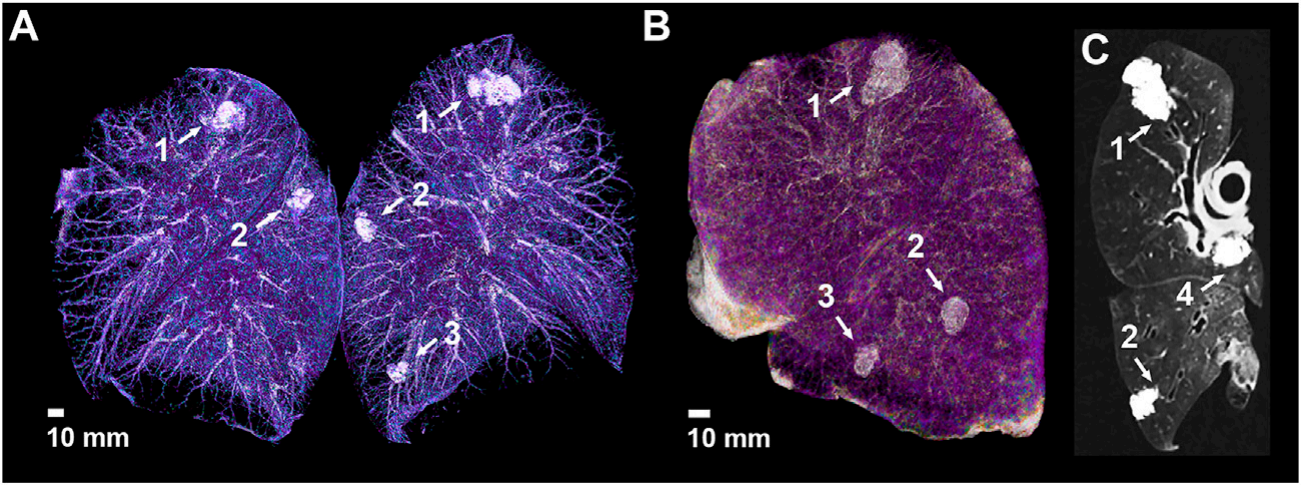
CT imaging of the isolated lungs implanted the artificial tumors. (A) Volume rendering of the CT scan with artificial tumors in different locations of both lungs; (B) Anterolateral view of a left lung showing three of the implanted artificial tumors; (C) Oblique CT slide of the left lung showing the fourth hilar lesion– 1: apical lesion, 2: 1.0 ml peripheral lesion, 3: 0.5 ml peripheral lesion, 4: hilar lesion.
